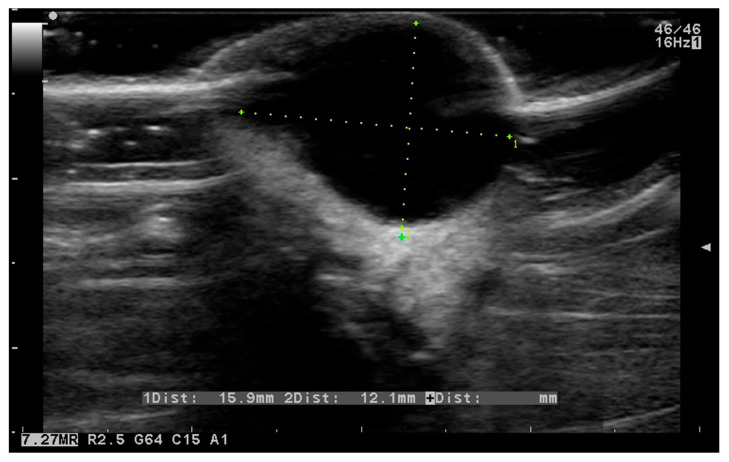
Longitudinal ultrasound view of the retroauricular lesion, demonstrating a well-circumscribed, anechoic, compressible cystic structure. Aloka prosound alpha 6, linear probe. Green caliper lines indicate the ultrasound measurement of the lesion.
